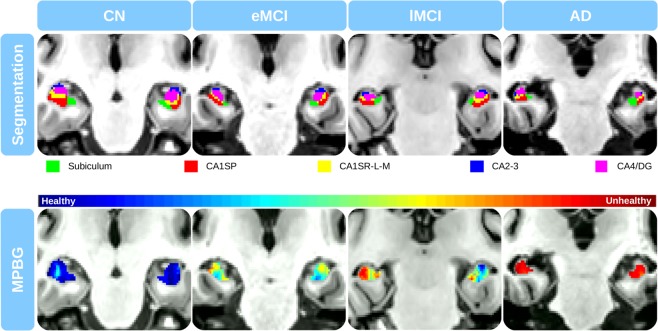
The presented results have been obtained using MRI from patients suffering from different severities of cognitive impairments. From top to bottom slices on the coronal plane of the segmentation maps, and the fusion of T1w and MD patch-based grading (*i*.*e*., MPBG: Multimodal Patch-Based Grading) with the proposed multimodal patch-based grading method. The blue and red colors represent healthy and altered tissues, respectively. To avoid bias due to overlap between training and testing datasets, the library has been constructed within a leave-one-out procedure.
